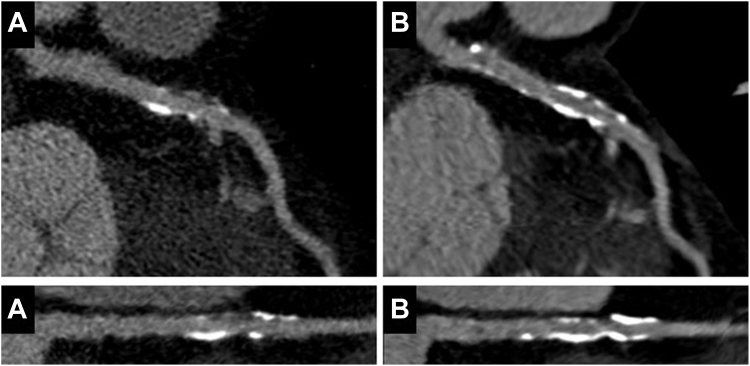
Serial CCTA with initiation of statin therapy after baseline coronary computed tomography angiography. Example of plaque on (A) baseline and (B) follow-up of the proximal left anterior descending artery in a patient with coronary artery disease. Statin therapy is started after the baseline coronary computed tomography angiography. The follow-up CT demonstrates progression of mainly calcified plaque.
